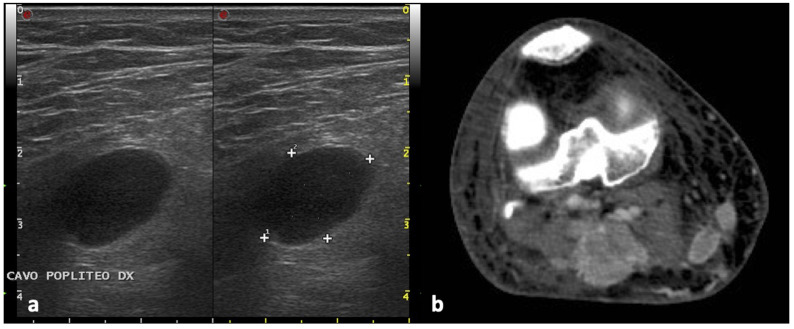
Lymphadenopathy of the right popliteal fossa from cutaneous melanoma of the calf. The B-Mode US scan (a) shows an oval, heterogeneous, predominantly hypoechoic lymph node with sharp margins. The contrast-enhanced CT axial-scan image (b) demonstrates a partially necrotic lymphadenopathy with peripheral enhancement.
